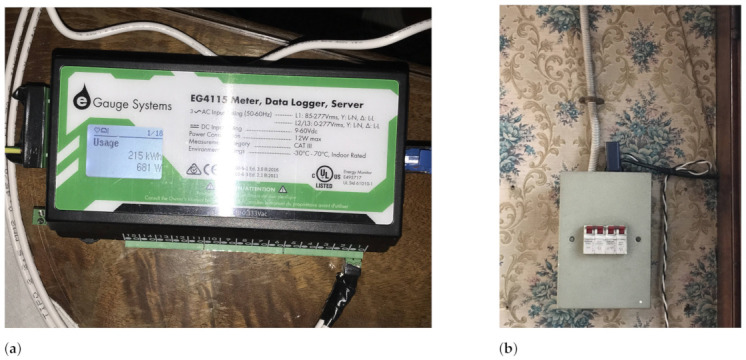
eGauge EG4115 data logger used as smart meter to collect energy consumption data from the main load of the house.(a) eGauge data logger unit showing instant power consumption; (b) CT Sensor (blue device) used to collect the current of the main load of the house.
